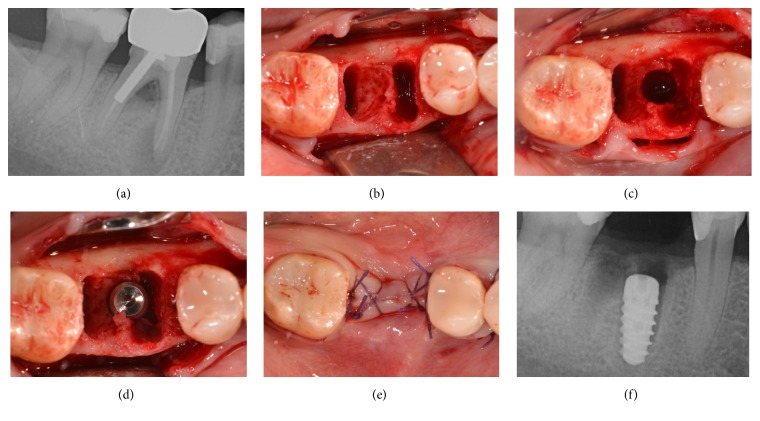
Immediate implant placement of a mandibular first molar (#46). Preoperative periapical radiograph before tooth extraction (a); postextraction socket and bone defect (b); implant site preparation in postextraction socket (c); implant placement in postextraction socket (d); primary flap closure and sutures (e); postoperative radiograph after implant surgery (f).
